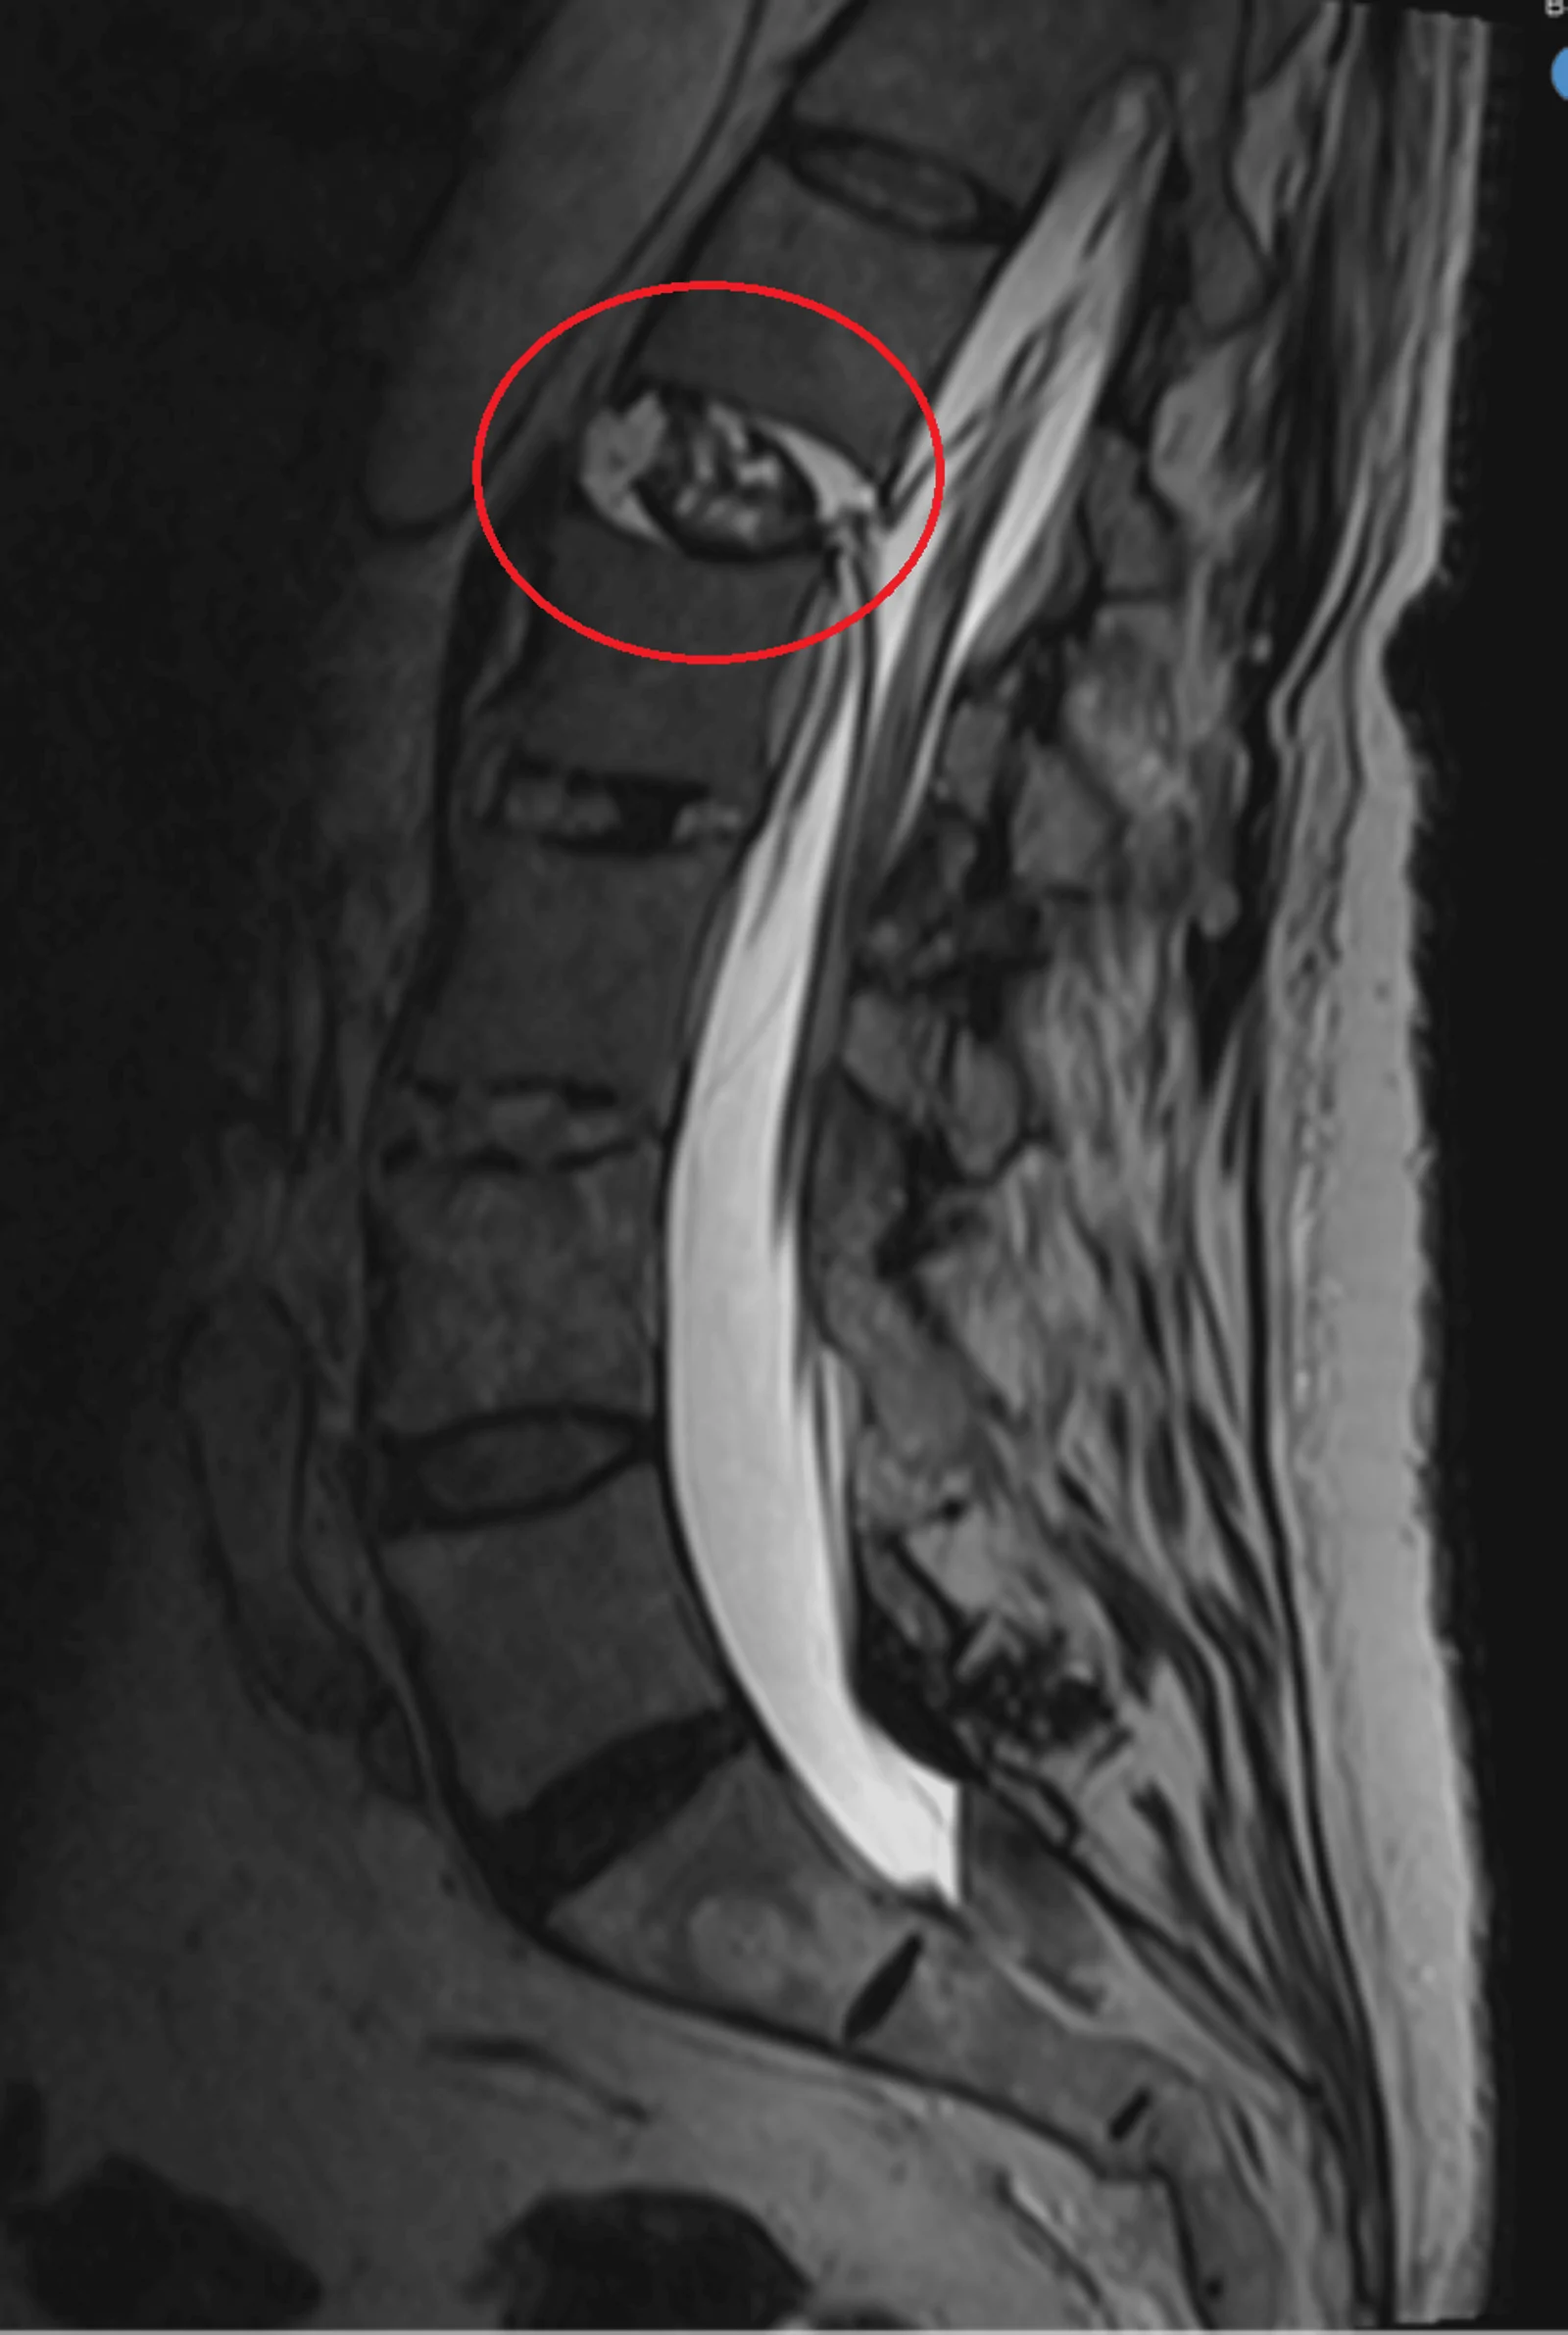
Sagittal T2-weighted MRI demonstrates disruption of the anterior and posterior longitudinal ligaments with entrapment of the anterior dural sac and ventral nerve roots within the fracture defect.
